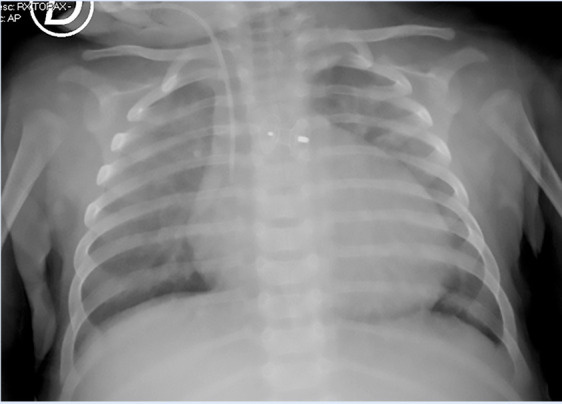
Post-procedural chest X-ray prior to discharge, showing the position of the occluder device, cardiomegaly, and pulmonary overcirculation.
